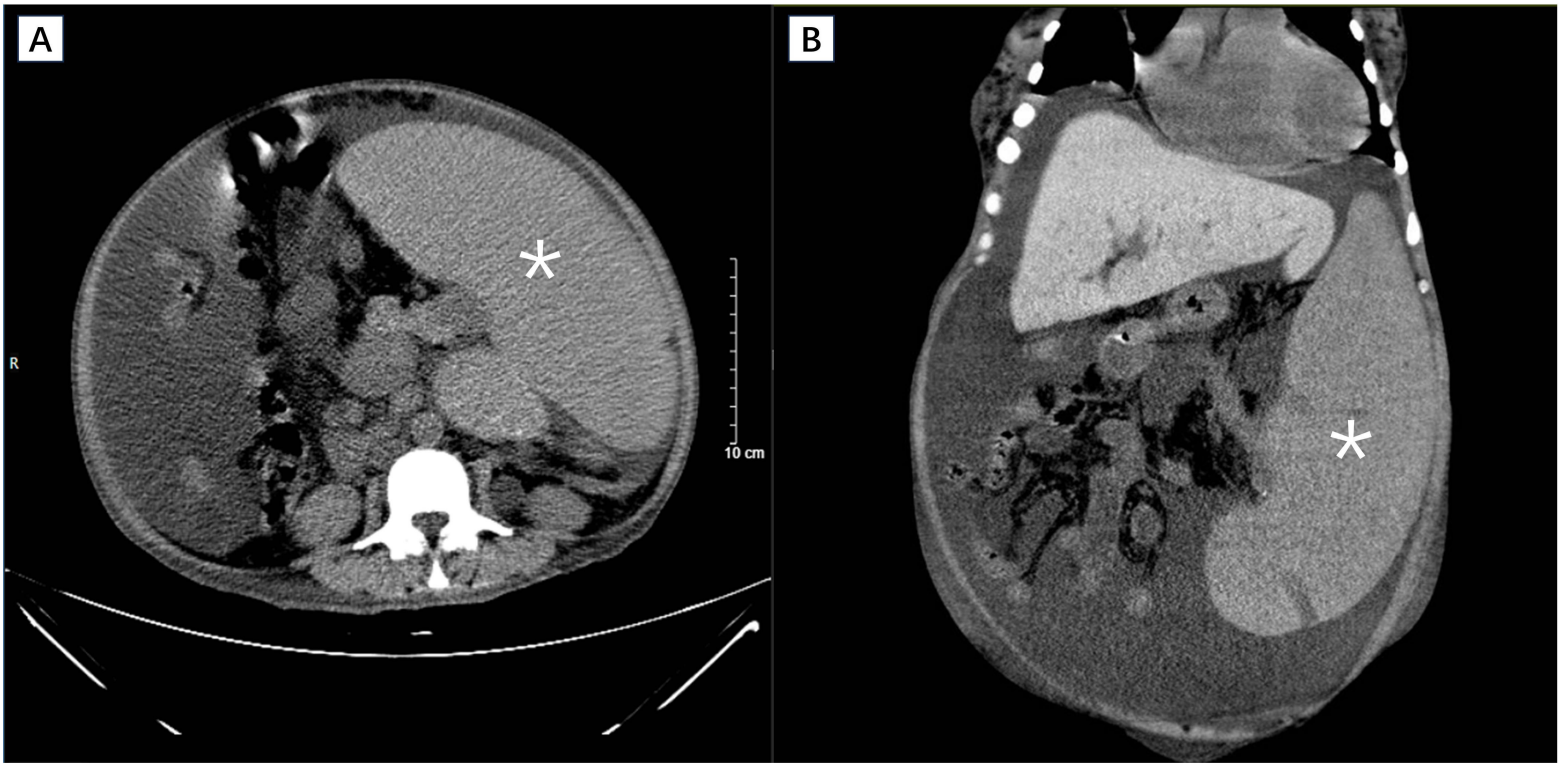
Cross sectional (A) and coronal (B) view of CT scan revealing massive splenomegaly (asterisk).
